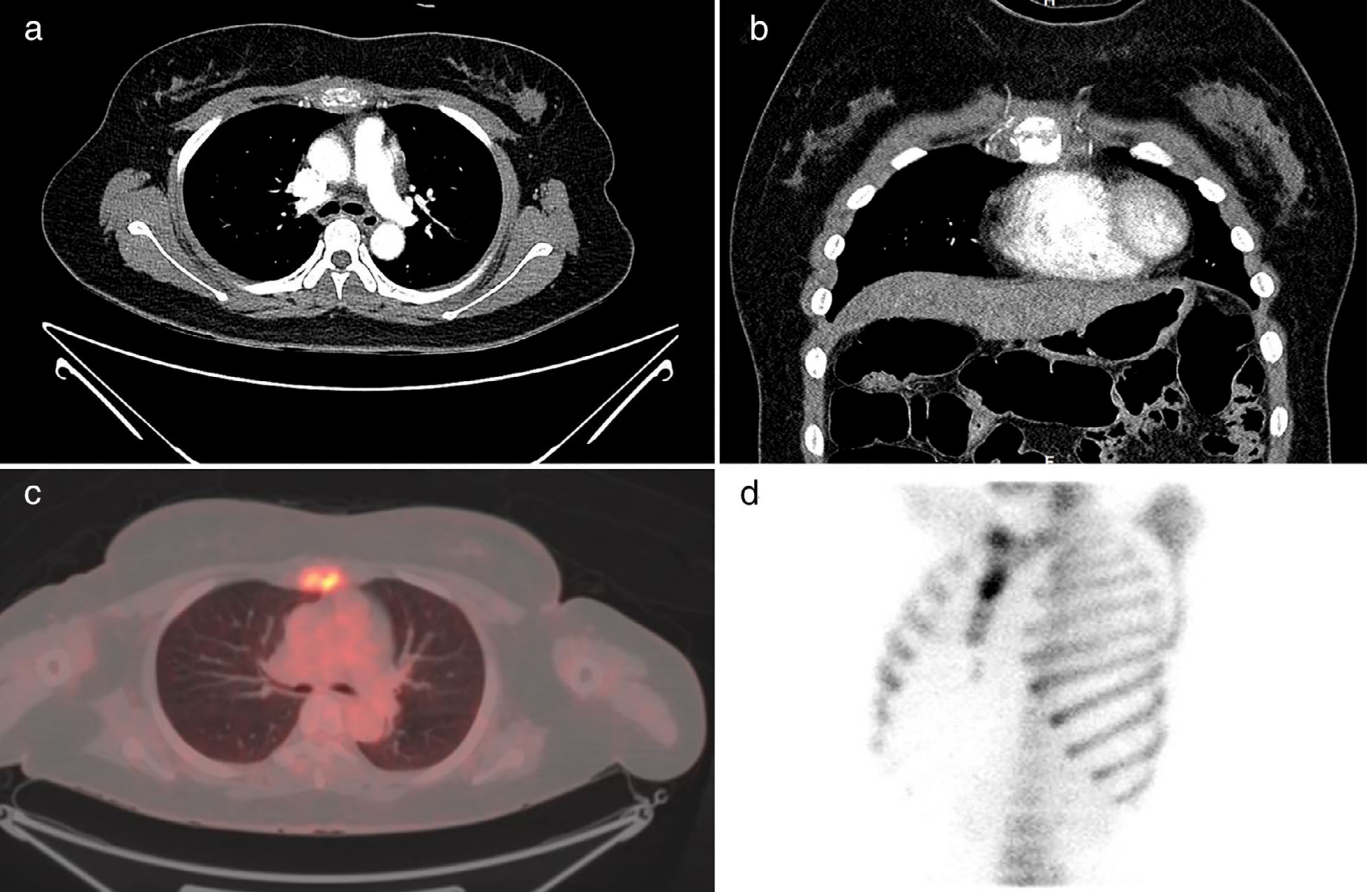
(a) Chest CT scan (axial view) showed a mass and a pathological fracture in the upper sternal body. (b) Chest CT scan (coronal view) showed a mass and a pathological fracture in the upper sternal body. (c) PET‐CT scan showed a heterogeneous hypermetabolic lytic bone lesion in the upper sternal body. (d) Bone scan showed increased focal uptake in the upper sternal body. CT, computed tomography; PET, positron emission tomography.
